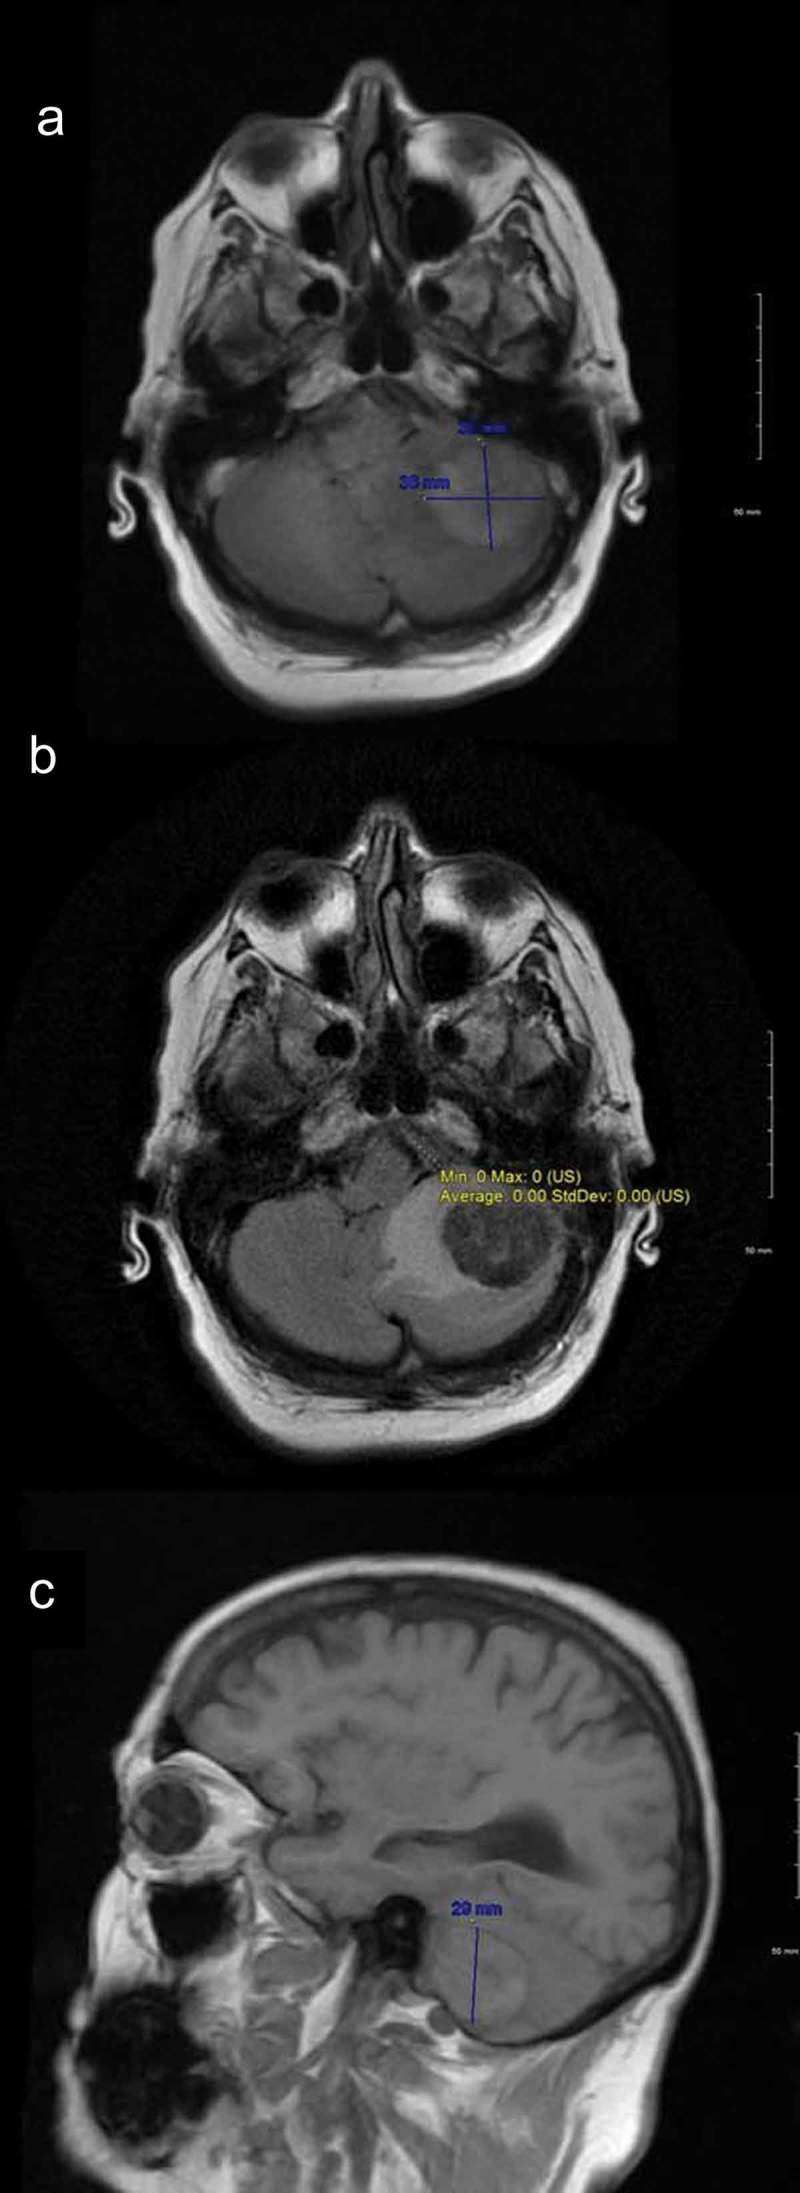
MRI brain showing left cerebellar lesion with significant amount of surrounding vasogenic edema, ipsilateral transtentorial herniation, and obliteration of the fourth ventricle.
